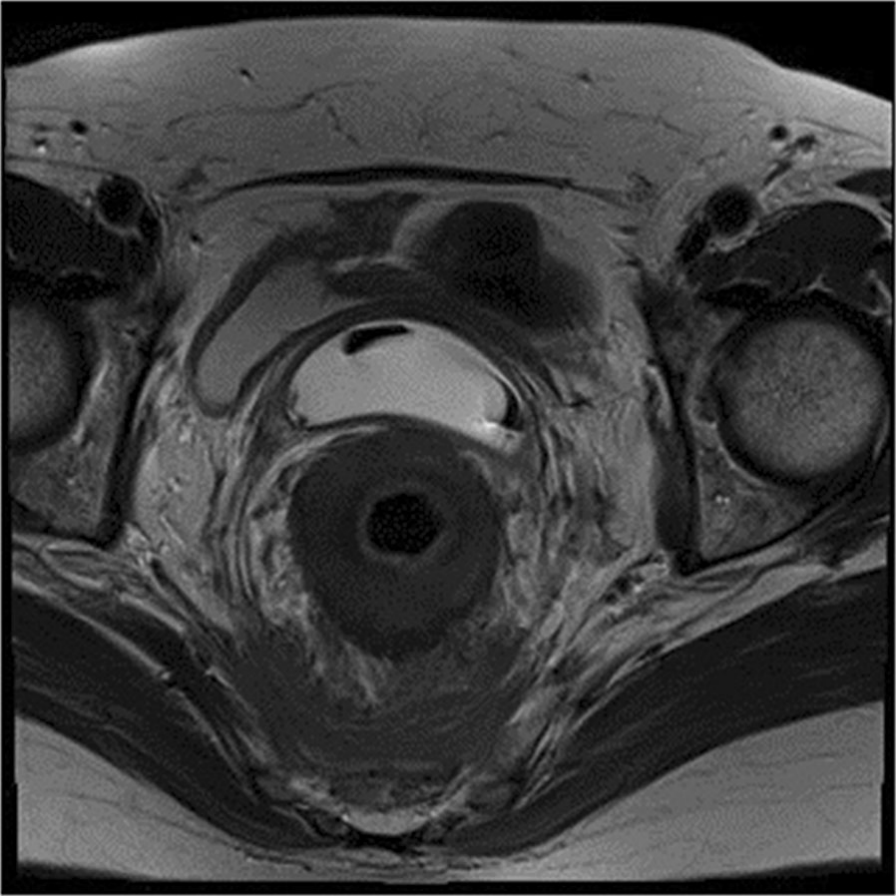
MRI shows diffuse wall thickening of the rectum and infiltration of the presacral fat tissue
